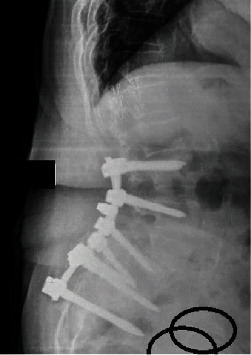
Preoperative lateral roentgenogram at one-year follow-up. L3 to lumbopelvic fixation.
